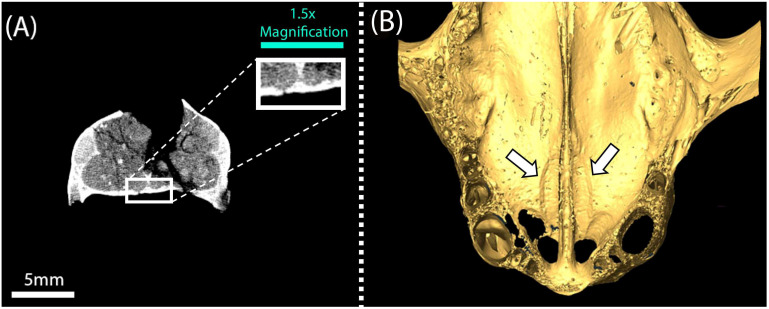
(A) A two-dimensional CT scan (slide 64) shows a coronal section of the cranium of *Mioeuoticus shipmani* (KNM-RU 2052) at the level of P_2_ with a vomeronasal groove on the base of the olfactory cavity. The boxed area of the image is magnified 1.5× in the top-right corner for comparison and clarity. (B) Dorsal view of the base of the olfactory cavity of *Perodicticus ibeanus* (AMNH-52682) with a pair of vomeronasal grooves. Vomeronasal grooves are indicated with white arrows. White scale bar is 5 mm. Blue scale bar at 1.5× magnification is 3.3 mm.
